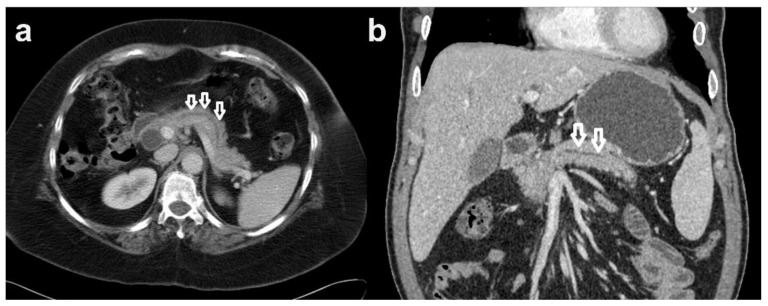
Pancreatic duct dilatation on CT scan. (a) CT scan - axial view of pancreatic duct dilatation. (b) CT scan - coronal view of pancreatic duct dilatation.
